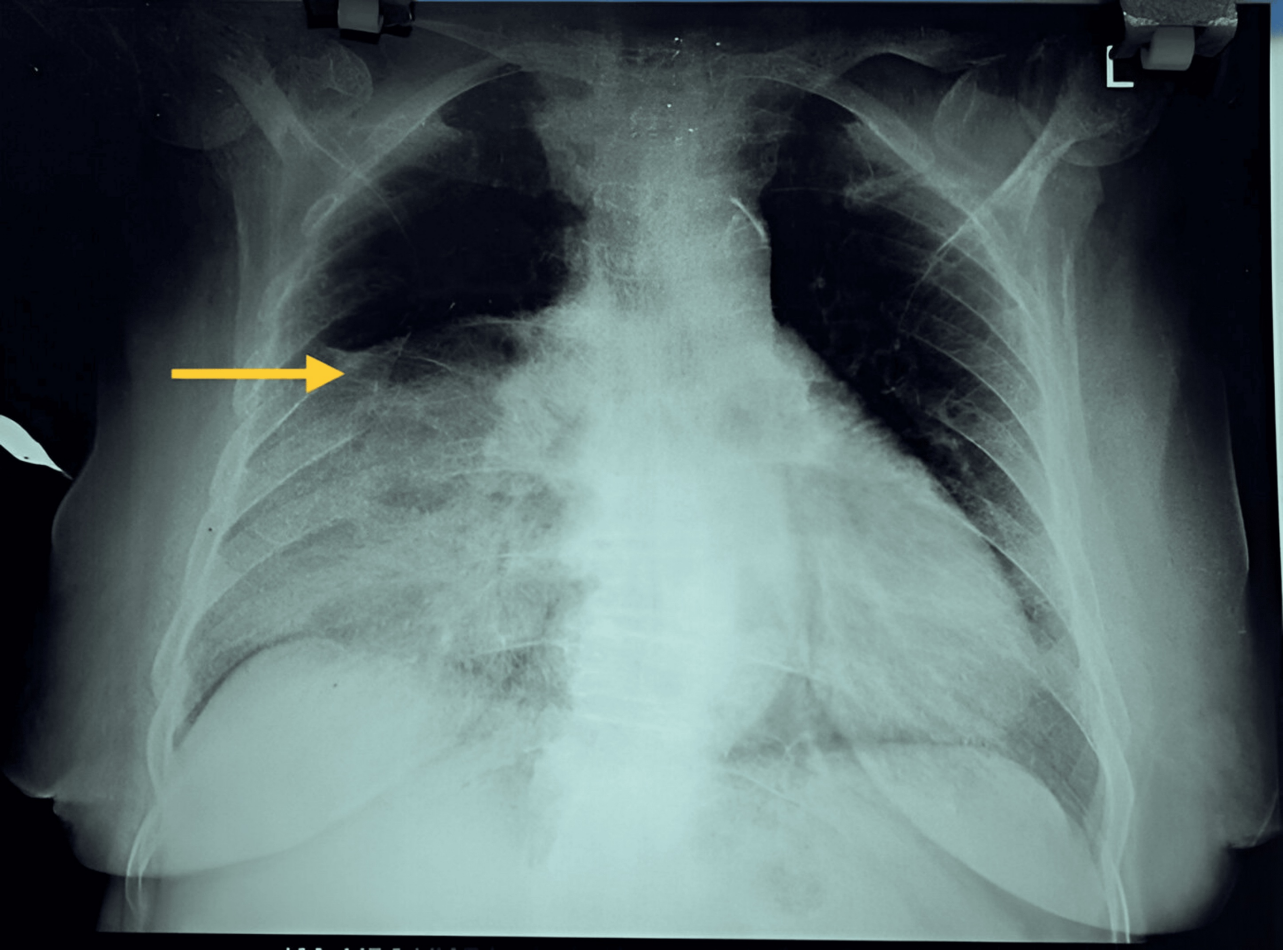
Chest X-ray showing non-homogeneous opacity in the right inframammary region, lower limit of visualized right lung parenchyma (yellow arrow), and mild mediastinal shift to the left side.
